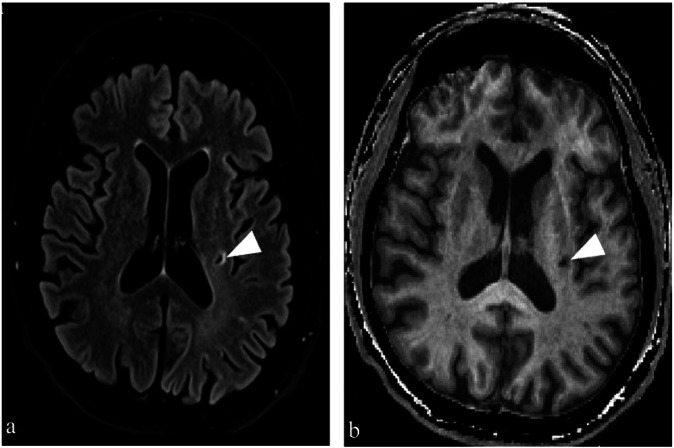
Representative case of a recovered COVID-19 patient with MRI-detectable macro- and microstructural brain changes. The 66-year-old male subject was hospitalized for over 3 months due to COVID-19. The MRI protocol included a conventional FLAIR sequence (a) and a synthetic anatomical MP-RAGE sequence (b), the latter of which was also used for software-based automatic segmentation of multiple deep gray matter structures. The visually interpreted images (a, b) revealed a lacuna in the left dorsal putamen (white arrowheads), which was excluded from the qT1 measurements (threshold exceeded). MRI qT1 maps showed increased qT1 values predominantly in the left caudate and accumbens nuclei (subject’s median qT1: 1,563 ms and 1,818 ms *versus* median qT1 of the never-infected controls aged ≥ 40: 1,398 ms and 1,644 ms), without any apparent lesions on conventional images. COVID-19, Coronavirus disease 2019; FLAIR, Fluid-attenuated inversion recovery; MP-RAGE, Magnetization-prepared rapid acquisition gradient-echo; MRI, Magnetic resonance imaging; qT1, Quantitative T1 relaxation times
